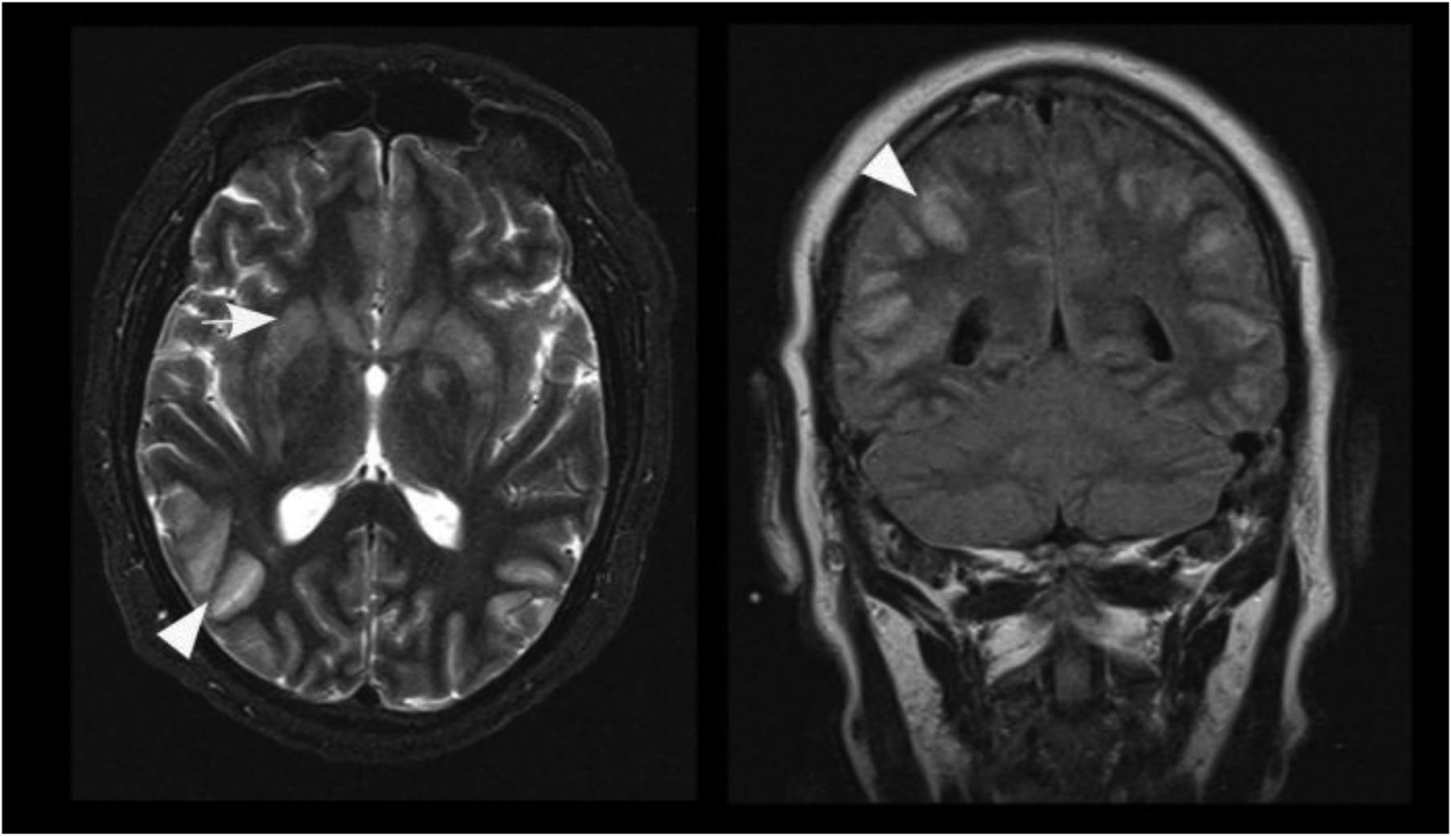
Axial T2 weighted image (left) and coronal FLAIR image (right) of patient B on day 44. Prominent cortical hyperintensities and swelling of cortical grey matter (arrowheads) dominate over signal increase of the basal ganglia (arrow) in this patient.
